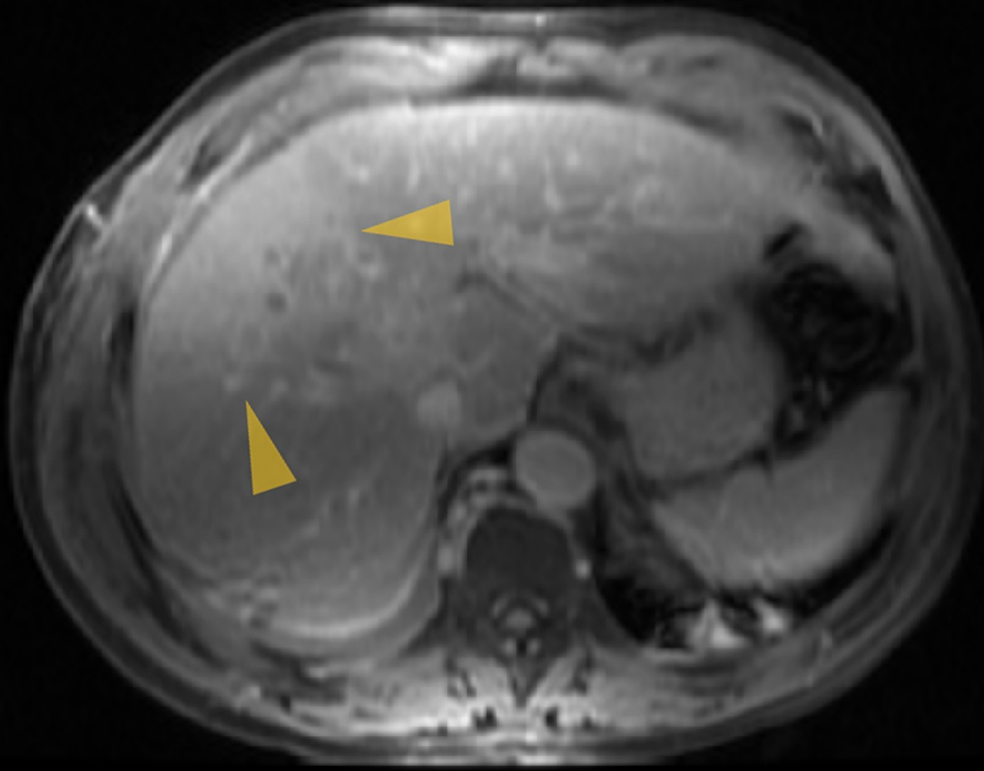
MRI abdomen with contrast showing hypervascular mass lesions with poorly defined margins in both the right and left lobes of the liver. The larger one measures 7 cm×5 cm in the anterolateral surface of the right lobe and abutting the diaphragmatic surface.
